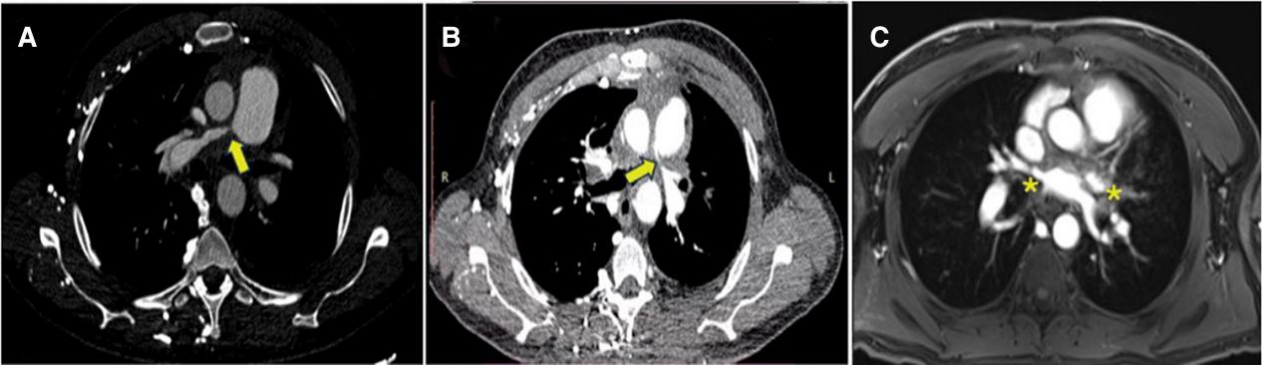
Angiotomography of great vessels and MRI of the thorax. Severe obstruction of the right (*A*) and left (arrows) (*B*) pulmonary arteries is evident. Chest magnetic resonance. (*C*) Scar tissue is observed in the superior mediastinum that involves branches of the right and left pulmonary arteries (asterisks).
